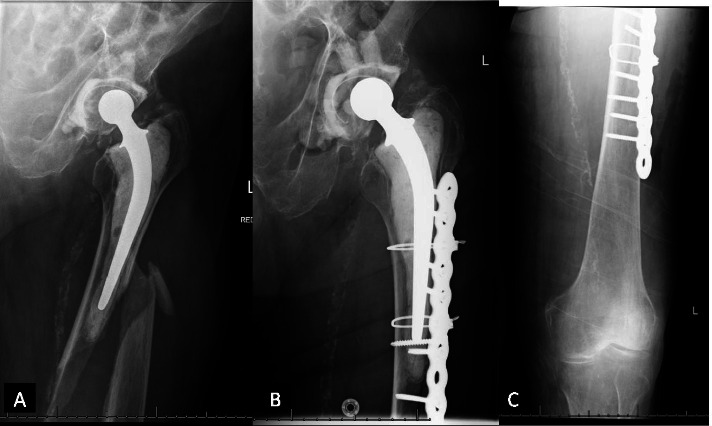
A periprosthetic femoral fracture (Vancouver type B1, left side). A Preoperative X-ray. B The X-ray taken 2 days after surgery shows the fracture is fixed with a cable plate system and locking screw. C The X-ray shows the middle portion of the femoral shaft
